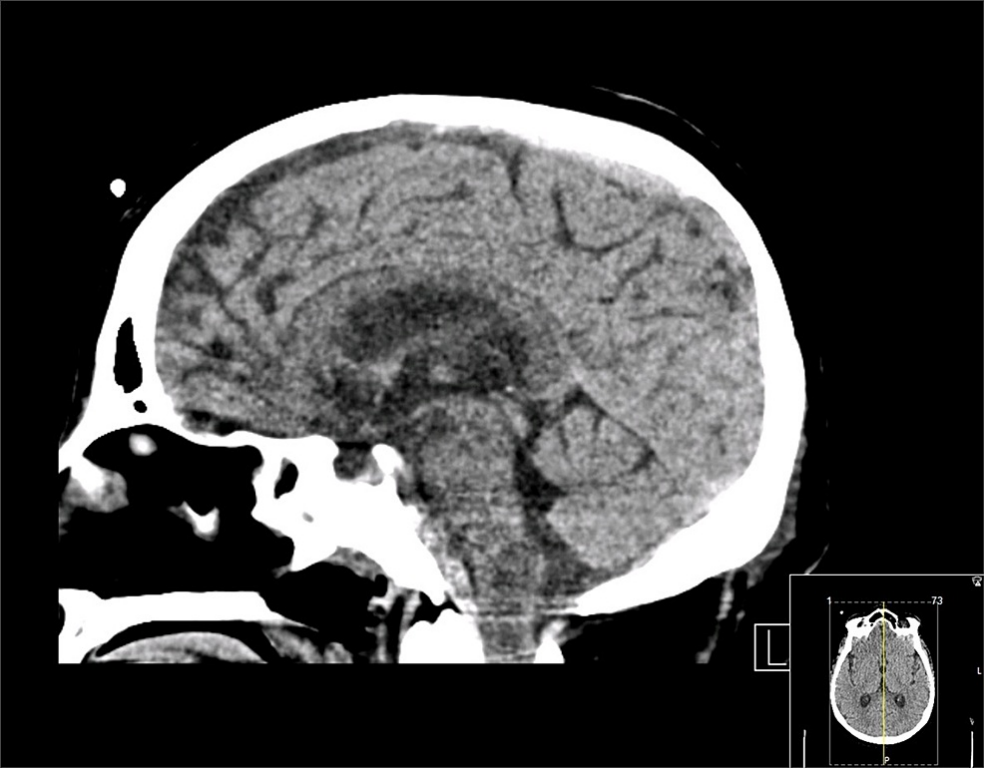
Sagittal brain CT scan revealing hypodensity of the lower brain stem
